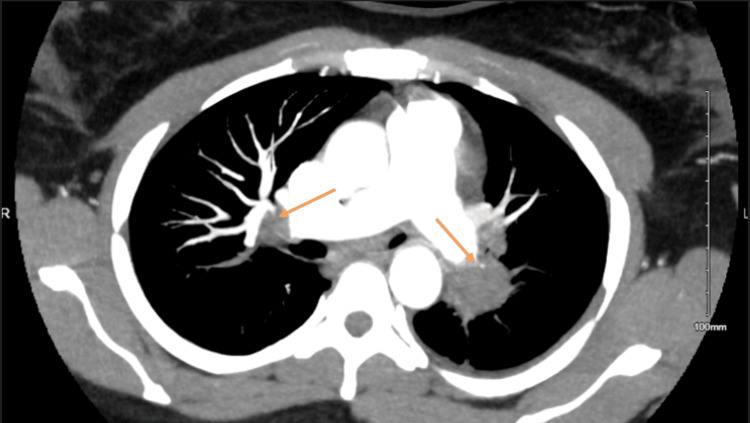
CT-PA demonstrating bilateral PE (orange arrows) CT-PA: computerized tomography of pulmonary arteries; PE: pulmonary embolism
